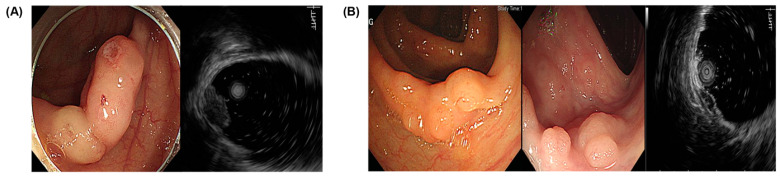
EUS findings of malignant lesions. (A) Adenocarcinoma and (B) lymphoma. EUS, endoscopic ultrasound.
